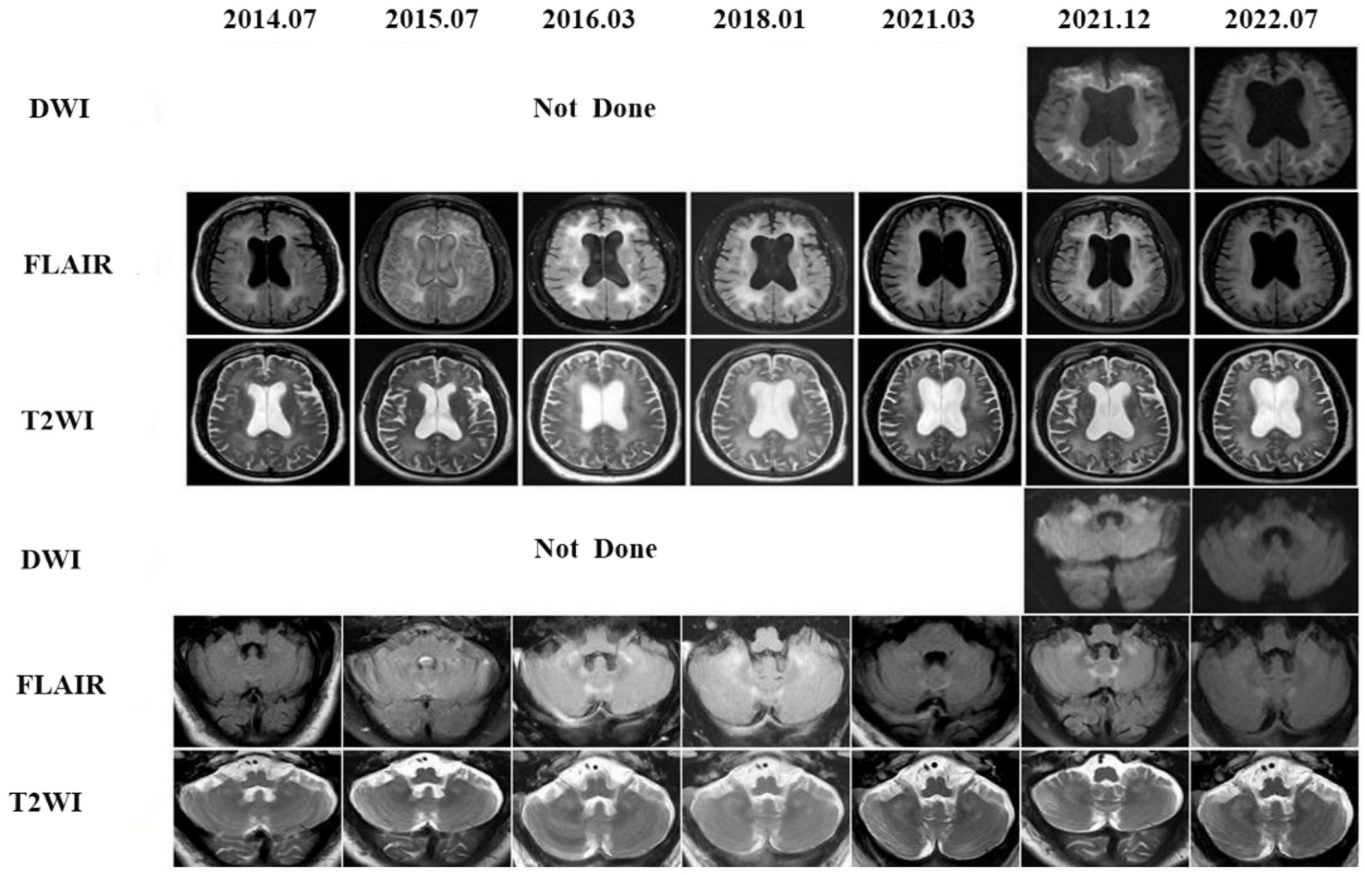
Eight years follow-up of the patient 3. The first brain DWI in our hospital was performed in 2021, high signal intensities in the CMJ, external capsules, the cerebellar white matter and paravermal regions on DWI already existed and kept stable after 1 year later. In fact, a series of conventional brain MRI images of this patient were undertaken from 2014 to 2021, showing leucoencephalopathy in those regions on FLAIR. Unfortunately DWIs were not done and the patient was misdiagnosed all the time until 2021.
